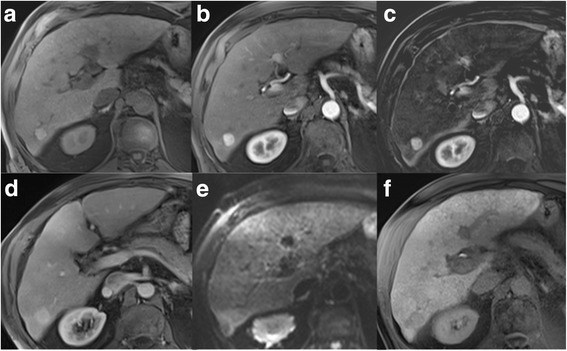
LR4. a Unenhanced T1w GRE image reveals a 17 mm nodule in the right lobe, which is b hypervascular in the arterial phase, best seen c in the subtraction image. d There is no washout present in the venous phase. e Ancillary features favouring malignancy are restricted diffusion in DWI and f hypointensity in hepatobiliary phase. According to size and hypervascularity without washout, lesion would be categorized as LR-3, but ancillary features justify upgrade to LR-4. Biopsy revealed HCC
